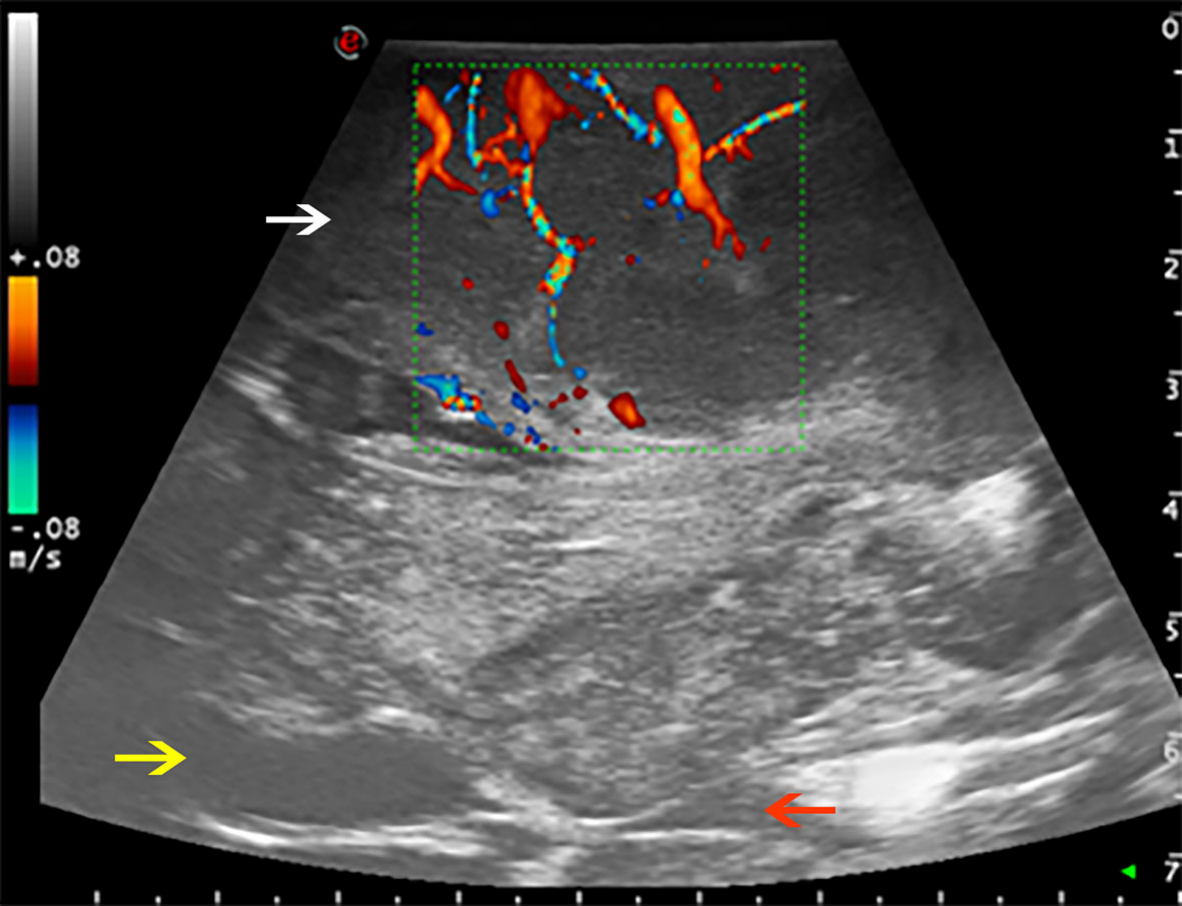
Color Doppler used to evaluate the perfusion pattern of a fronto-temporo-parietal GBM (case no. 6). White arrow: glioblastoma. Yellow arrow: frontal horn of lateral ventricle. Red arrow: third ventricle.
